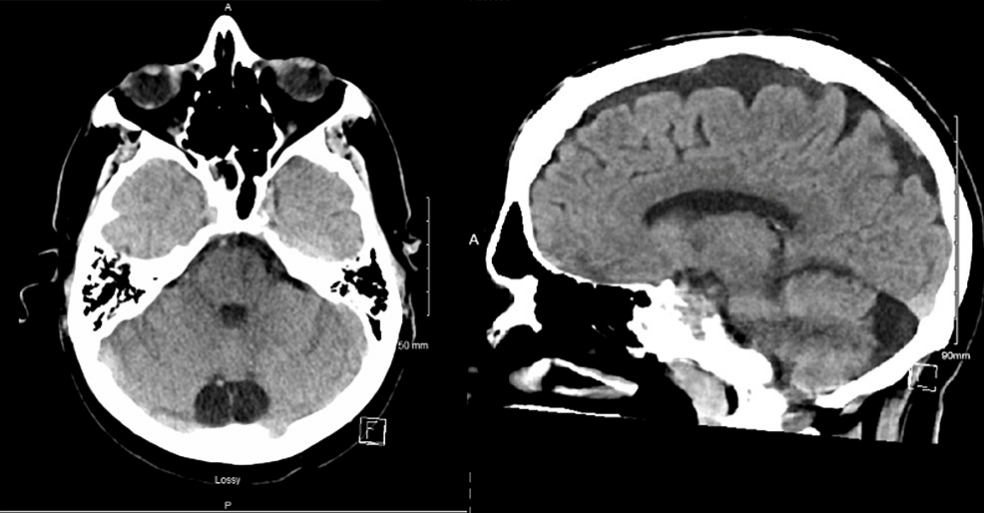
CT Brain without contrast with no signs of acute intracranial hemorrhage, mass effect, midline shift or herniation and an incidental finding on cysterna magna.
